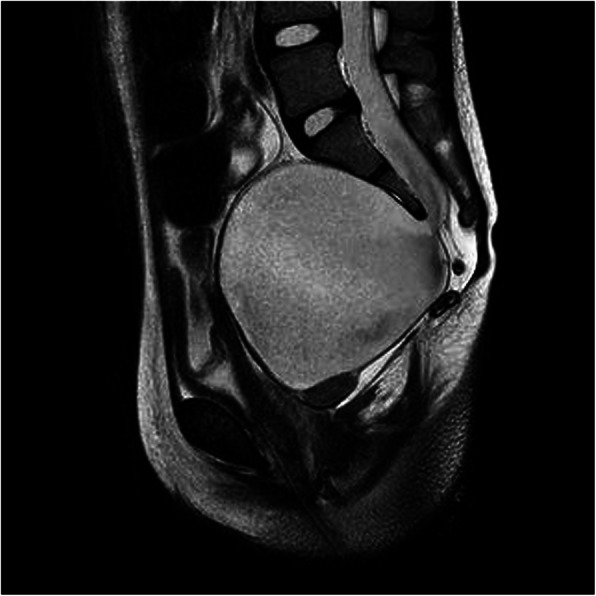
T2W MRI sagittal image showing anterior sacral meningocele (8.1 × 8.1 × 8.2 cm) and underlying teratoma (19 mm). The latter was T1W-hyperintense, and T2W- and STIR- hypointense, showing no contrast-enhancing
